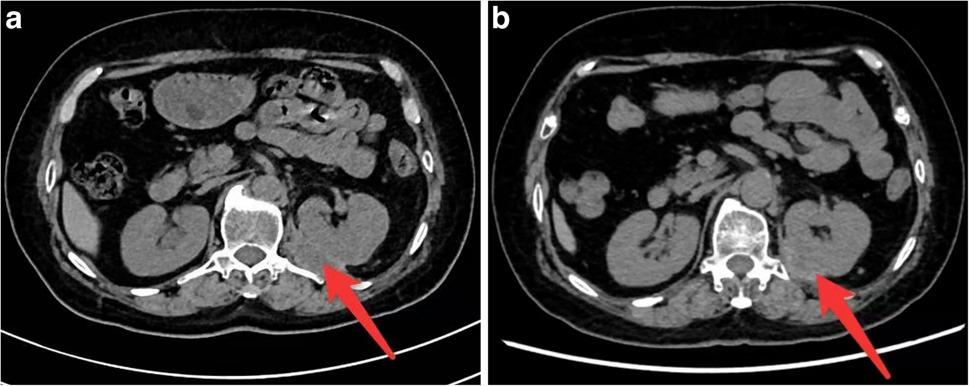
a CT image obtained on the day before discharge (hospital day 30) demonstrates a perirenal hematoma (red arrow). b CT image obtained 30 days after discharge demonstrates resolution of the perirenal hematoma (red arrow)
